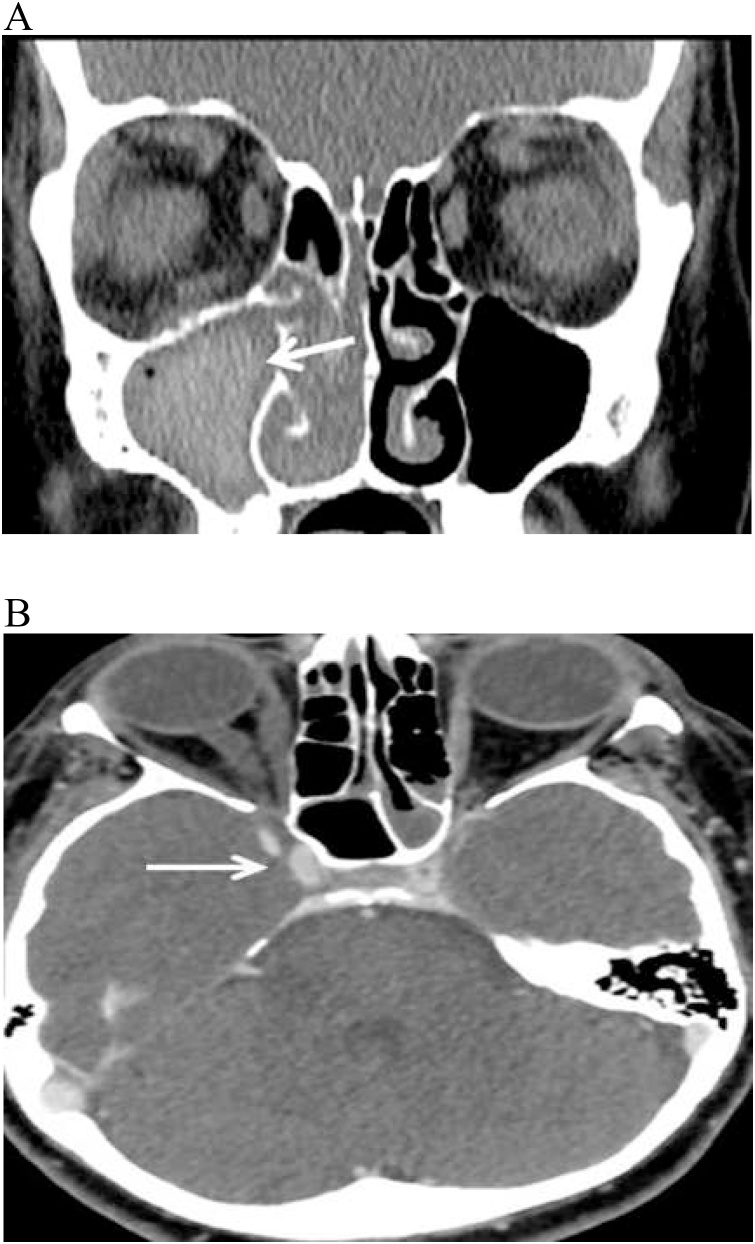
A: CT without contrast showing moderate mucosal thickening. B: CT venogram shows absence of enhancement of the right cavernous sinus.
